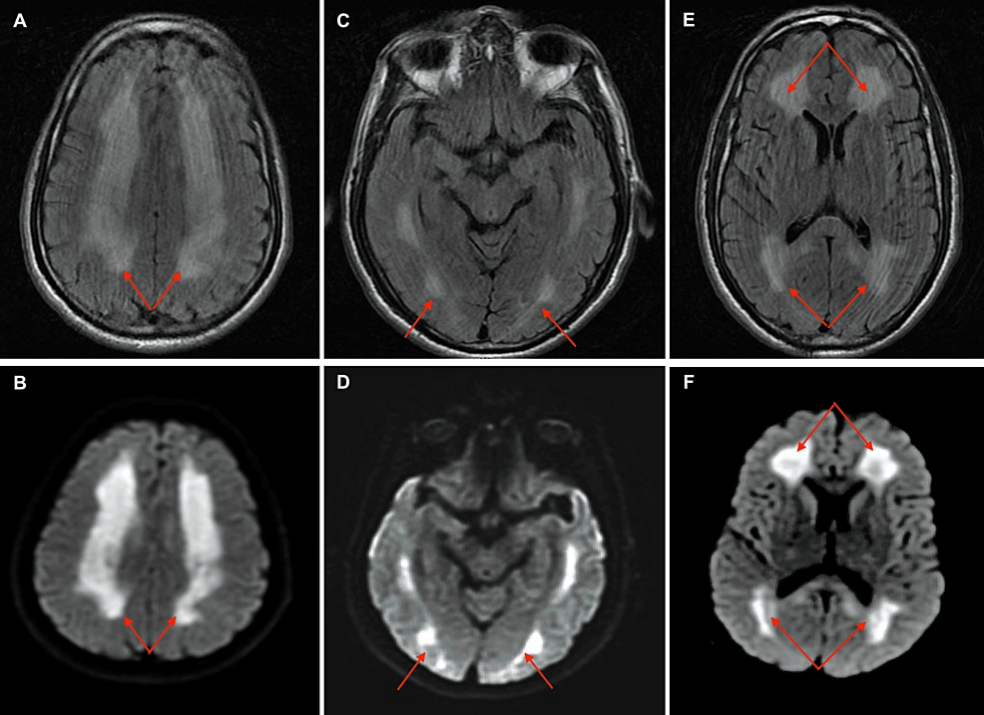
MRI brain showing T2 fluid-attenuated inversion recovery (FLAIR) signal hyperintensity with corresponding restricted diffusion throughout entire supratentorial white matter (red arrows) insult suggestive of multifocal leukoencephalopathy. No acute focal infarct, hemorrhage, mass effect, or hydrocephalus. Images A, C, and E represent T2 fluid-attenuated inversion recovery (FLAIR) hyperintensities over posterior frontal and parietal centrum semiovale, subcortical U fibers in the occipital lobe and white matter in basal ganglia respectively. Images B, D, and F demonstrate corresponding diffusion restriction on Diffusion-weighted imaging (DWI).
